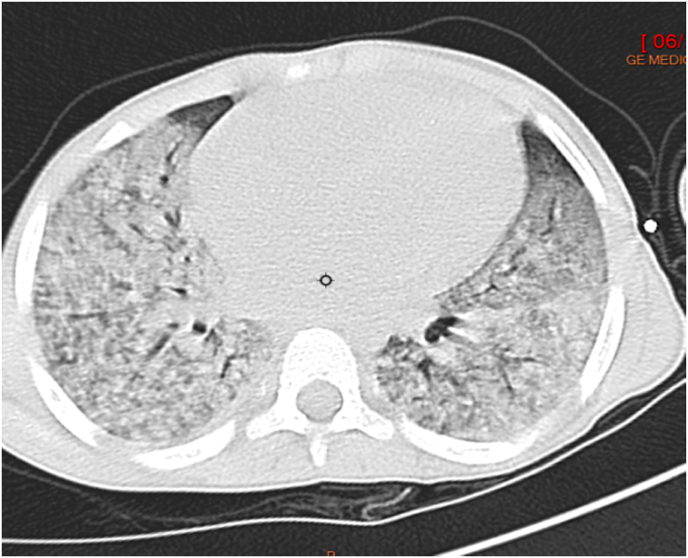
Thoracic CT scan showing lung damage of >90%.
